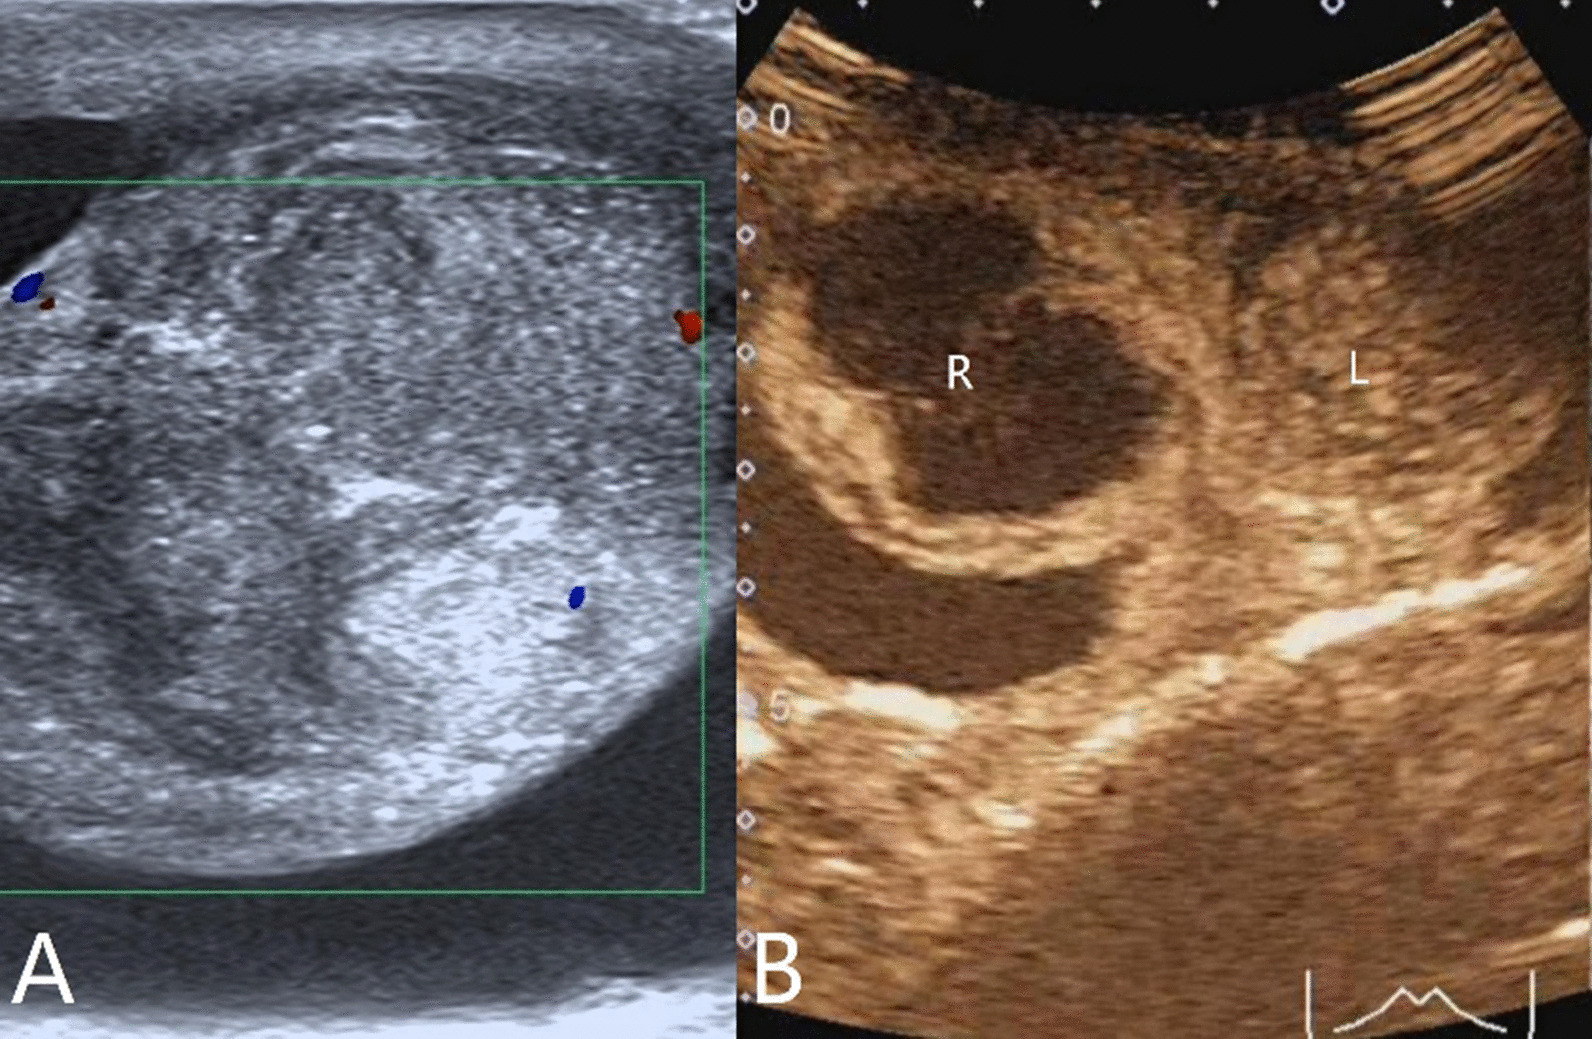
Testicular embryonal carcinoma. A Conventional ultrasonography showed that the right testis had an unclear boundary, irregularly shaped and uneven hypoechoic signals, and a little blood flow around it. B A large area of necrosis was evident in the center of the CEUS with high enhancement in the periphery. R: Right testis; L: left testis
